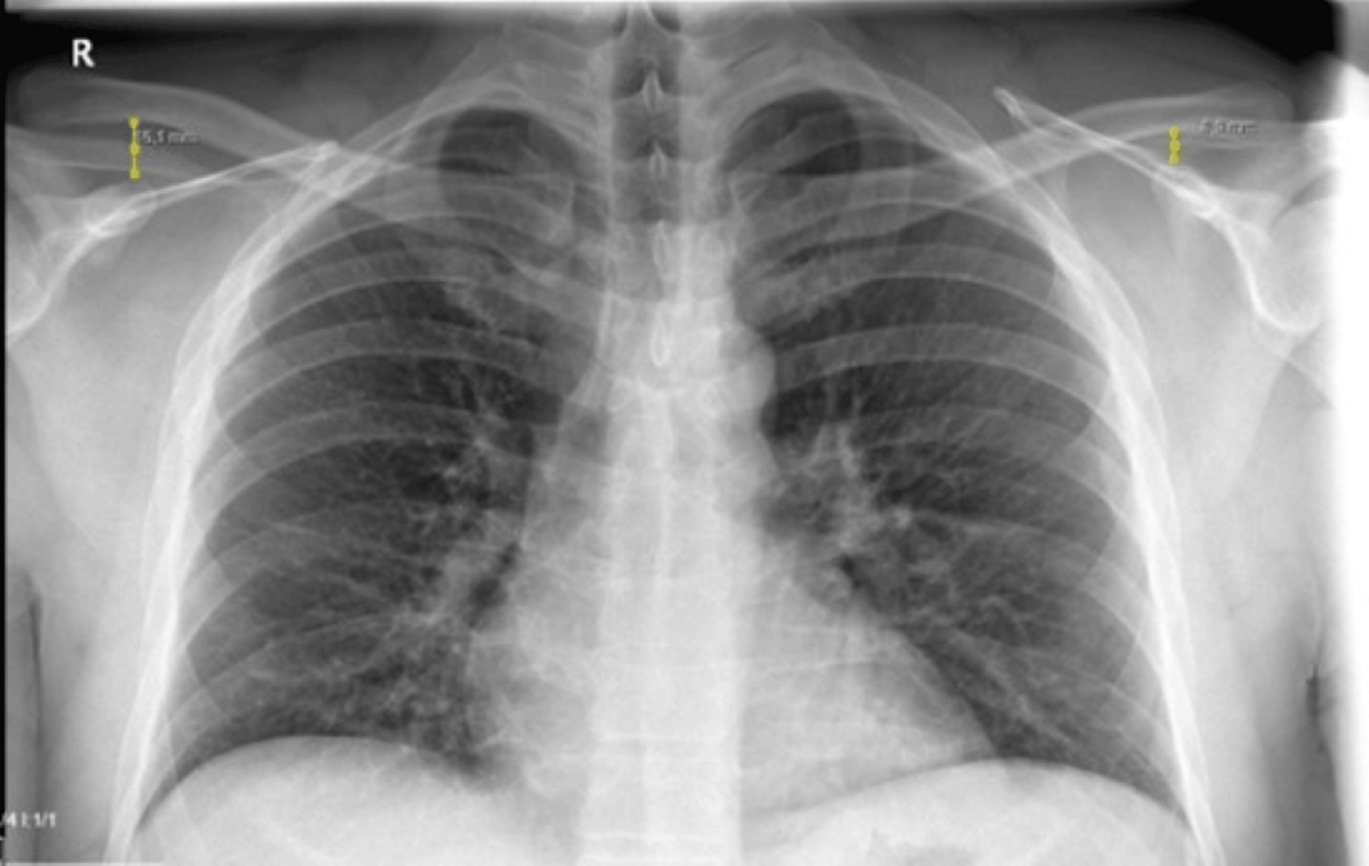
PA shoulder radiograph of the patient The image shows bilateral CC distance measurements: 16 mm on the right side and 8 mm on the left. The red arrows indicate the AC joint separation. AC, acromioclavicular; CC, coracoclavicular
